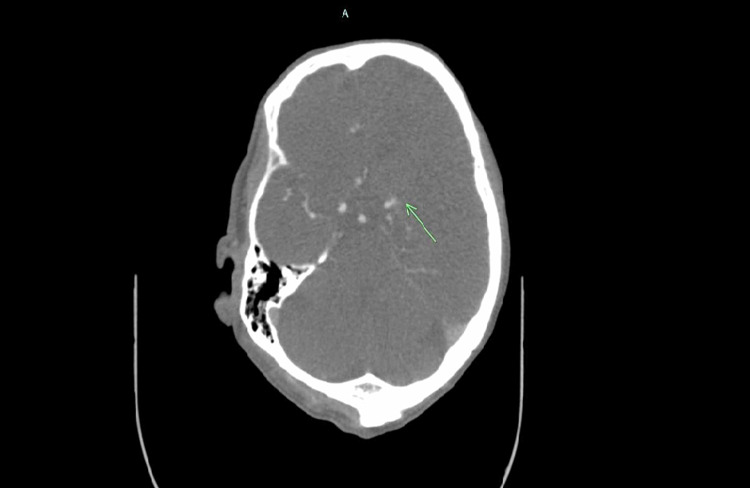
CT angiogram of the head with contrast The arrow indicates proximal left middle cerebral artery occlusion.
